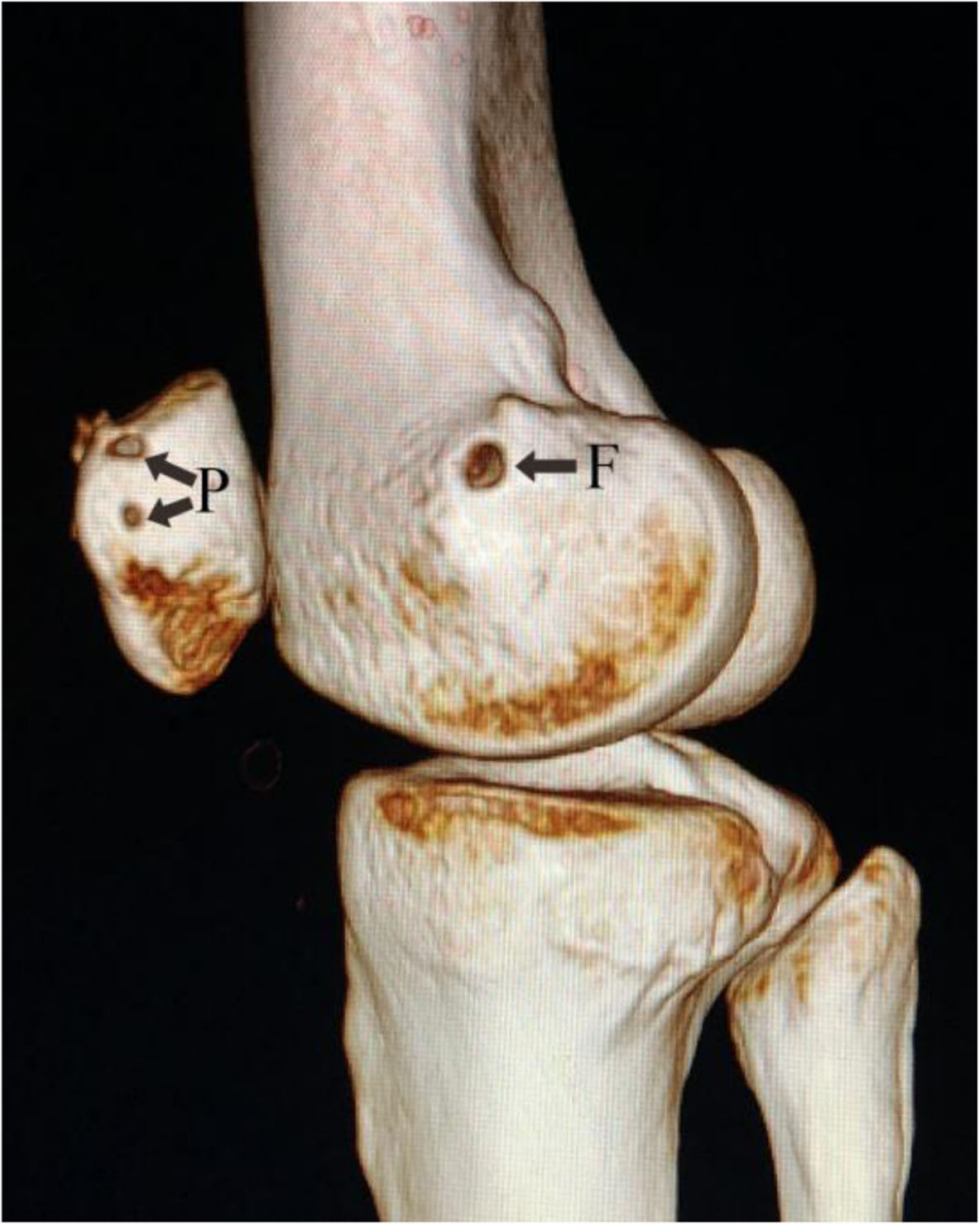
Postoperative three-dimensional CT showing the position of the bone tunnels. *F* femoral bone tunnel, *P* patellar bone tunnels
